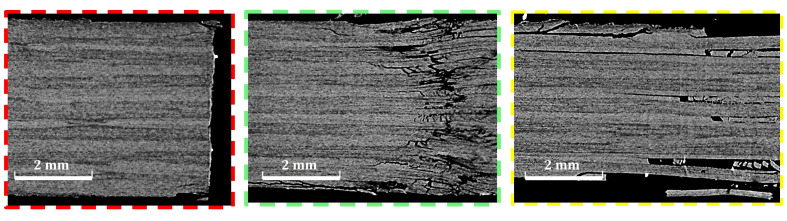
Computed Tomography scan of the second alternative (color code is in Figure 7).
